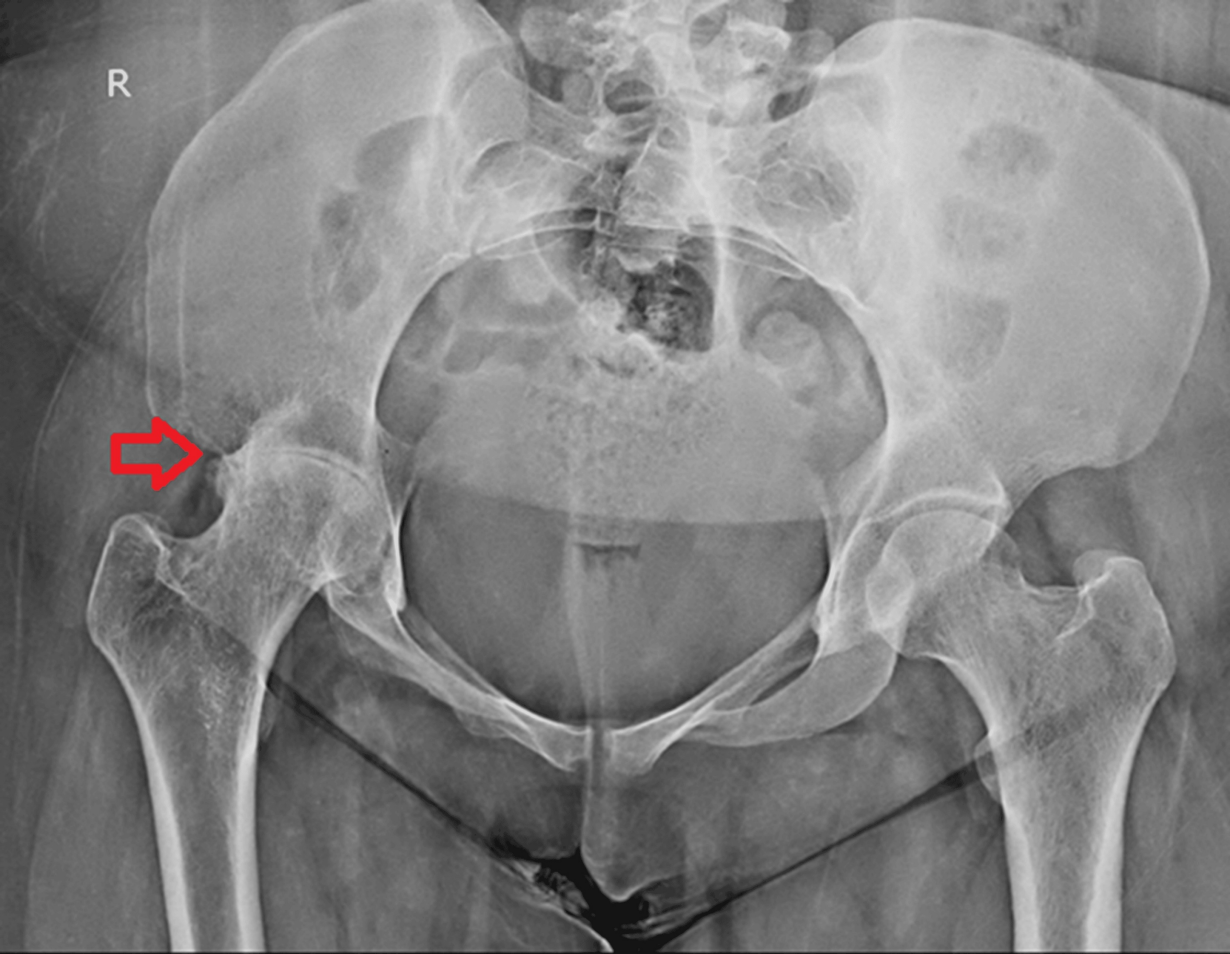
Follow-up radiograph after 12 months of ATT shows no further advancement of arthritic changes in the right hip joint. ATT: anti-tubercular therapy
